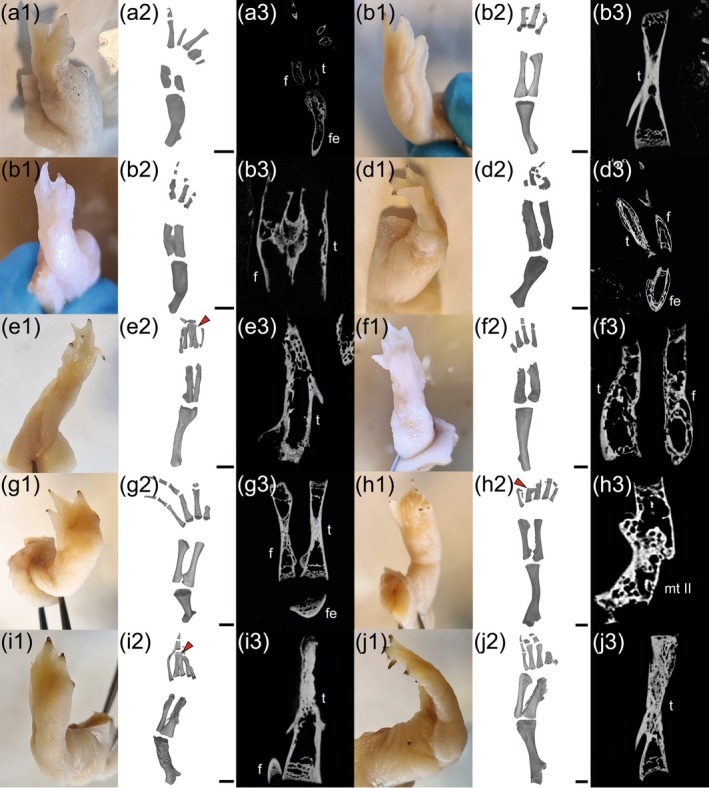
Internal limb skeletal anatomy after regeneration of adult *A. mexicanum*. Micro CT scans and 3D reconstructions of left (a, c, e, g, and i) and right hind limbs (b, d, f, h, and j) in an unstained condition. (a1–j1) External limb morphology. (a2–j2) Associated 3D models of unstained CT scans. (a3–j3) Associated longitudinal sections of selected areas. (a) Femur thickened in the distal area. Shortened and thickened long bones in the zeugopod. Significantly shortened digits caused by missing phalangeal elements. (b) Stylopod and zeugopod appear unregenerated. Incorrect digit anatomy including missing digits and phalangeal elements. (c) Femur thickened in the distal area. Thickened long bones in the zeugopod. Central fusion of tibia and fibula. Incorrect digit anatomy including missing digits and phalangeal elements. (d) Slightly thickened and irregularly shaped tibia and fibula. Incorrect digit anatomy including missing digits and phalangeal elements. (e) Distal fusion of metatarsal II and III (red arrow). Missing phalangeal elements in all digits. (f) Slightly thickened tibia and fibula. Incorrect digit anatomy including missing digits and phalangeal elements. (g) Stylopod, zeugopod, and digit I and II appear unregenerated. Incorrect digit anatomy including missing digits and phalangeal elements. Incorrect digit anatomy of digit III and IV caused by missing phalangeal elements. Digit 5 is completely absent. (h) Stylopod and zeugopod appear unregenerated. All digits shortened caused by missing phalangeal elements. Deformed metatarsal element (red arrow). (i) Thickened humerus. Irregular distal ends of tibia and fibula. Fusion of metacarpals III and IV (red arrow). Missing phalangeal elements in all digits. (j) Femur, fibula, and digit V appear unregenerated. Missing phalangeal elements in digits I–IV. f = fibula, fe = femur, mt = metatarsal, t = tibia. Scale bars represent 5 mm.
